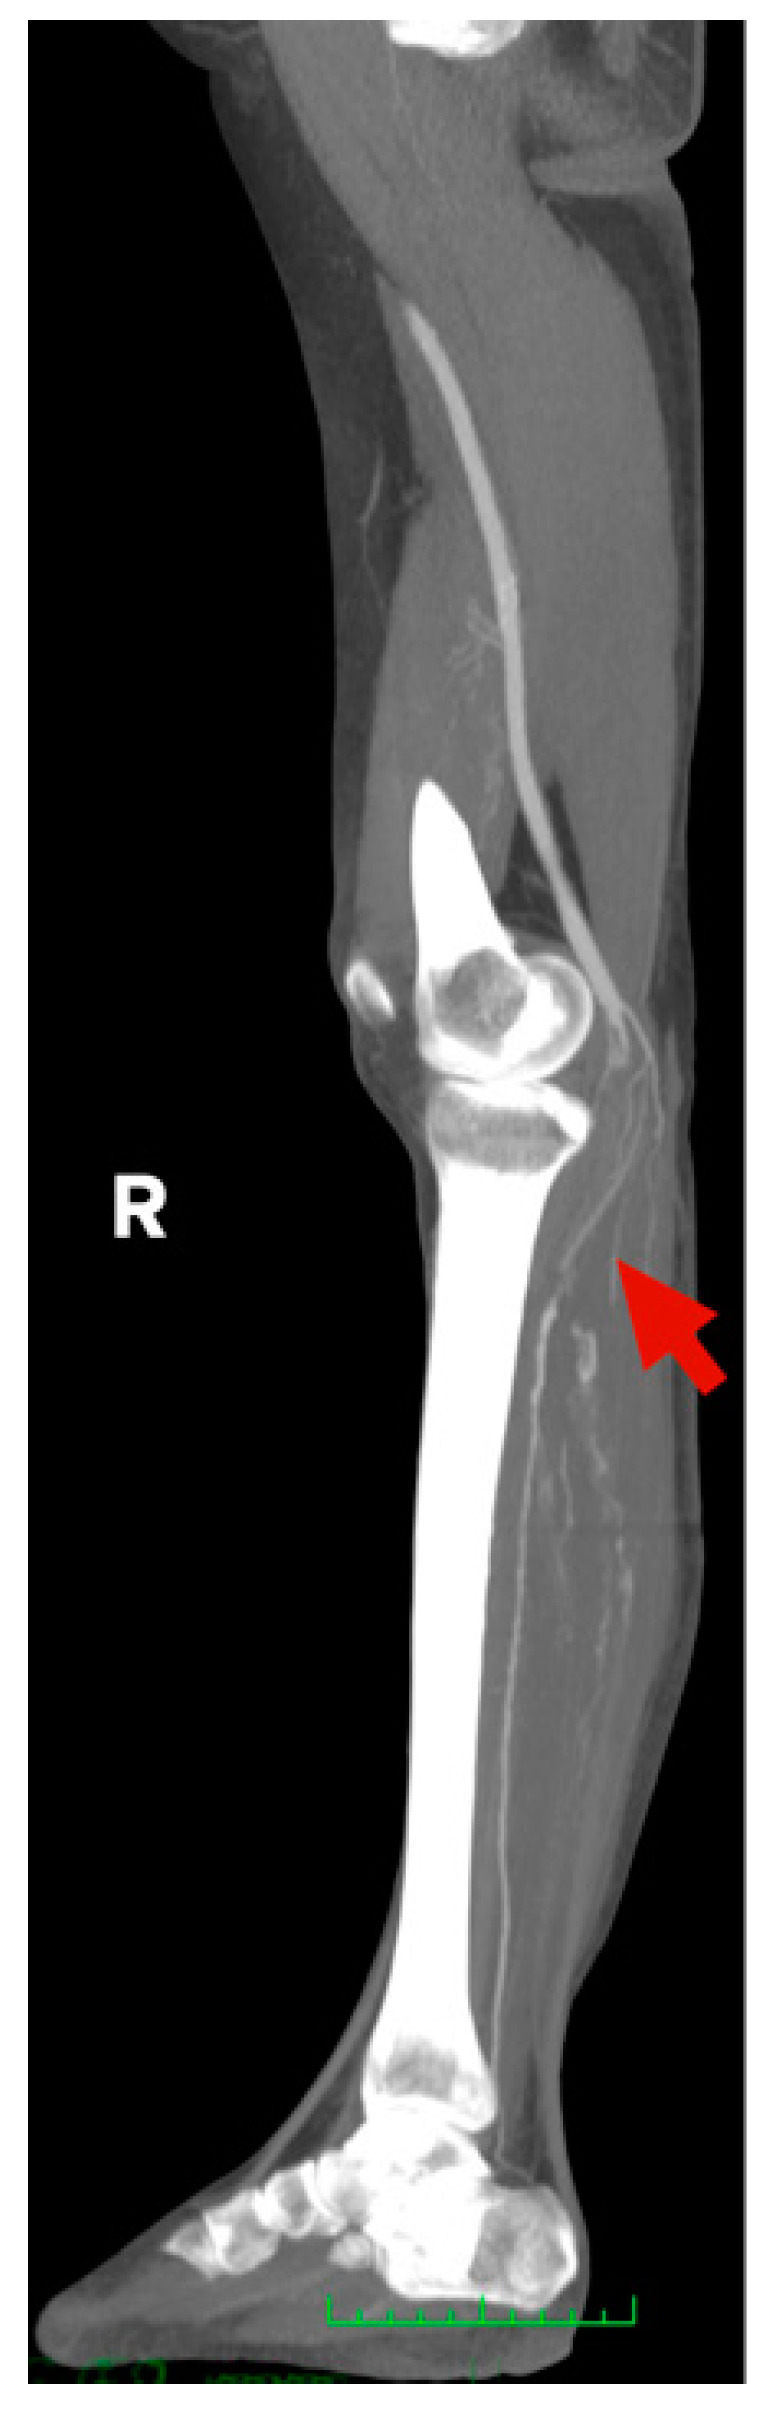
CT-Angio of 58 yo female with stage IIB acute right lower limb ischemia 14 days after SARS-CoV-2 infection.
